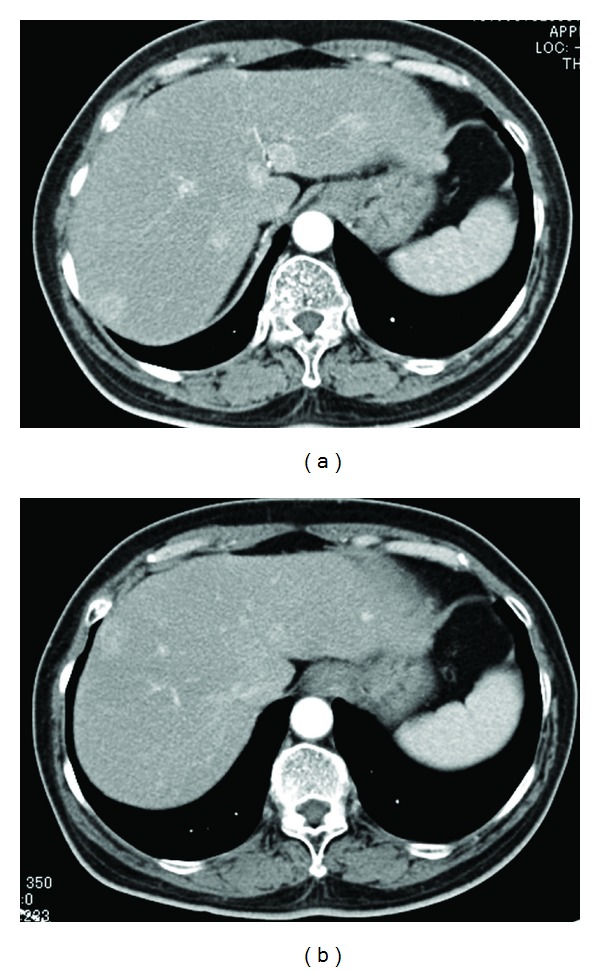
(a) Abdominal CT scanning after 24 months from surgical treatment showed multiple small nodules in the liver with no abnormal findings in other organs. (b) Multiple metastatic nodules have remarkably reduced after two cycles of hepatic arterial infusion of cisplatin.
